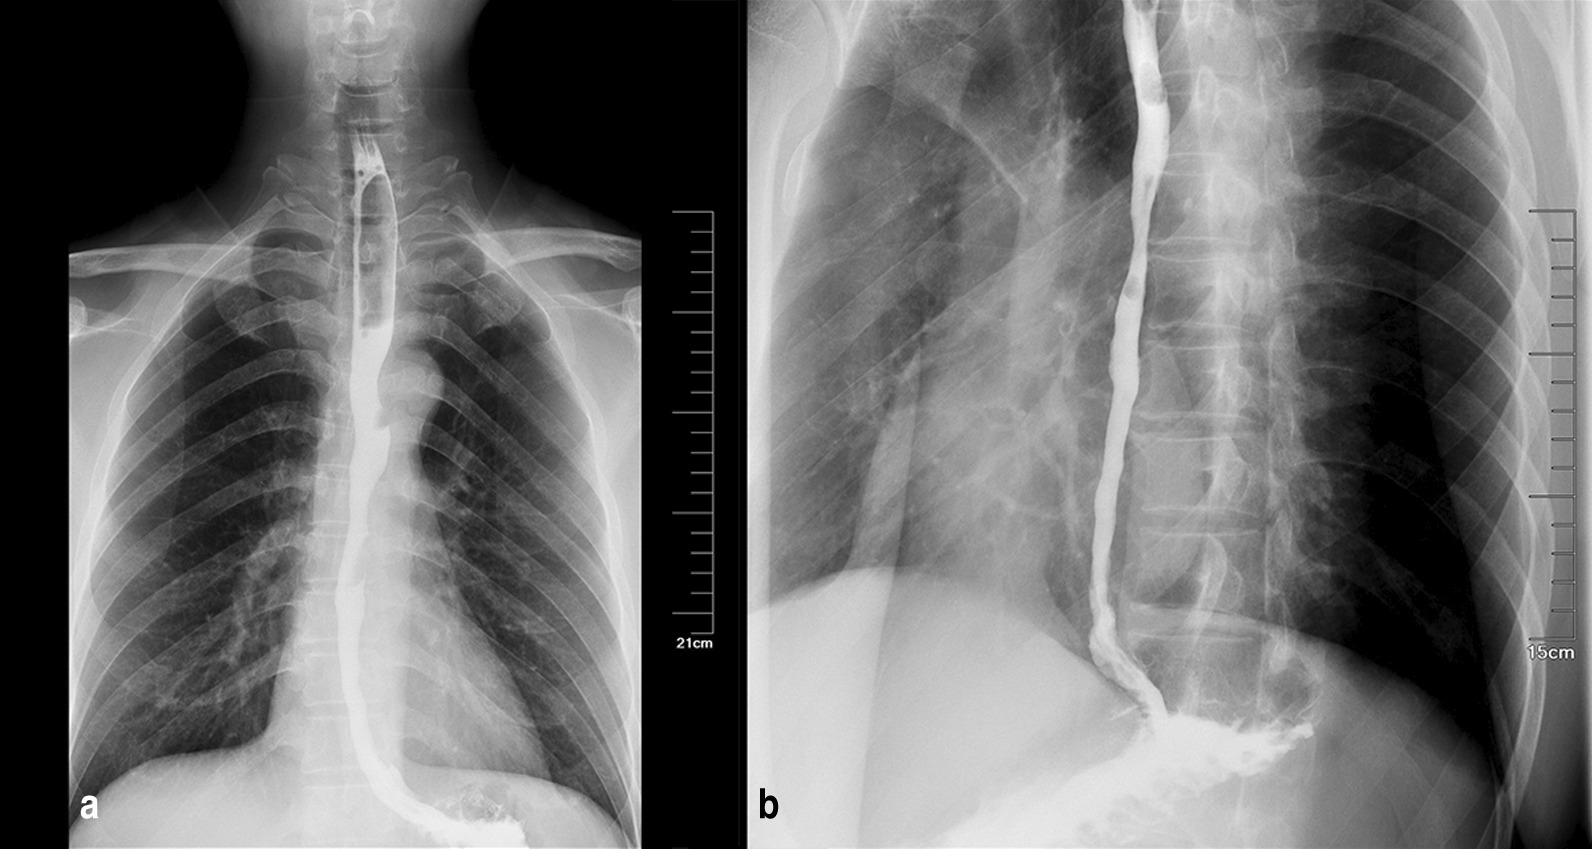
Barium esophagogram showed irregular mucosa changes along the distal esophagus on an anterior–posterior chest X-ray (a) and a lateral chest X-ray (b)
